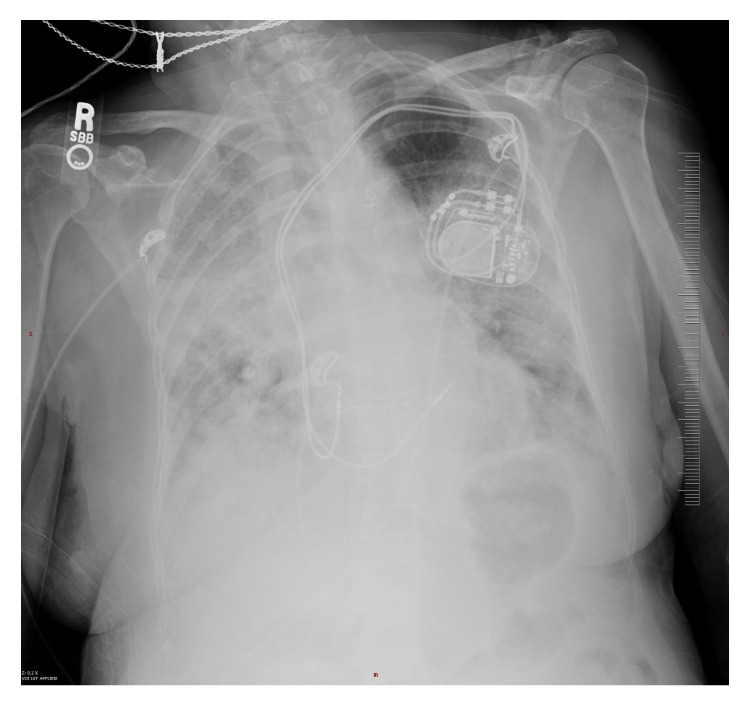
A chest X-ray, taken 5 days after the chest X-ray depicted in Figure 3, revealing rapid progression of lung infiltrates in multiple lobes.
